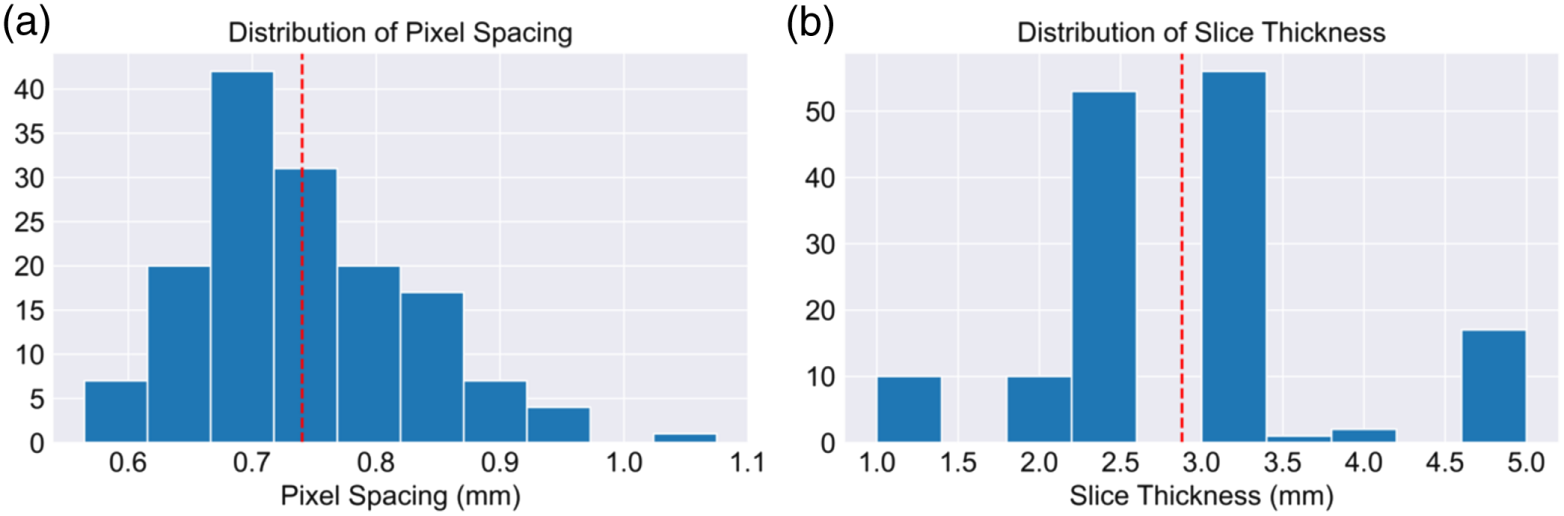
Histogram of the (a) pixel spacing and (b) slice thickness of CT sections of the original 149 scans. The red vertical line depicts the mean value in each of the distributions to which resampling was performed.
